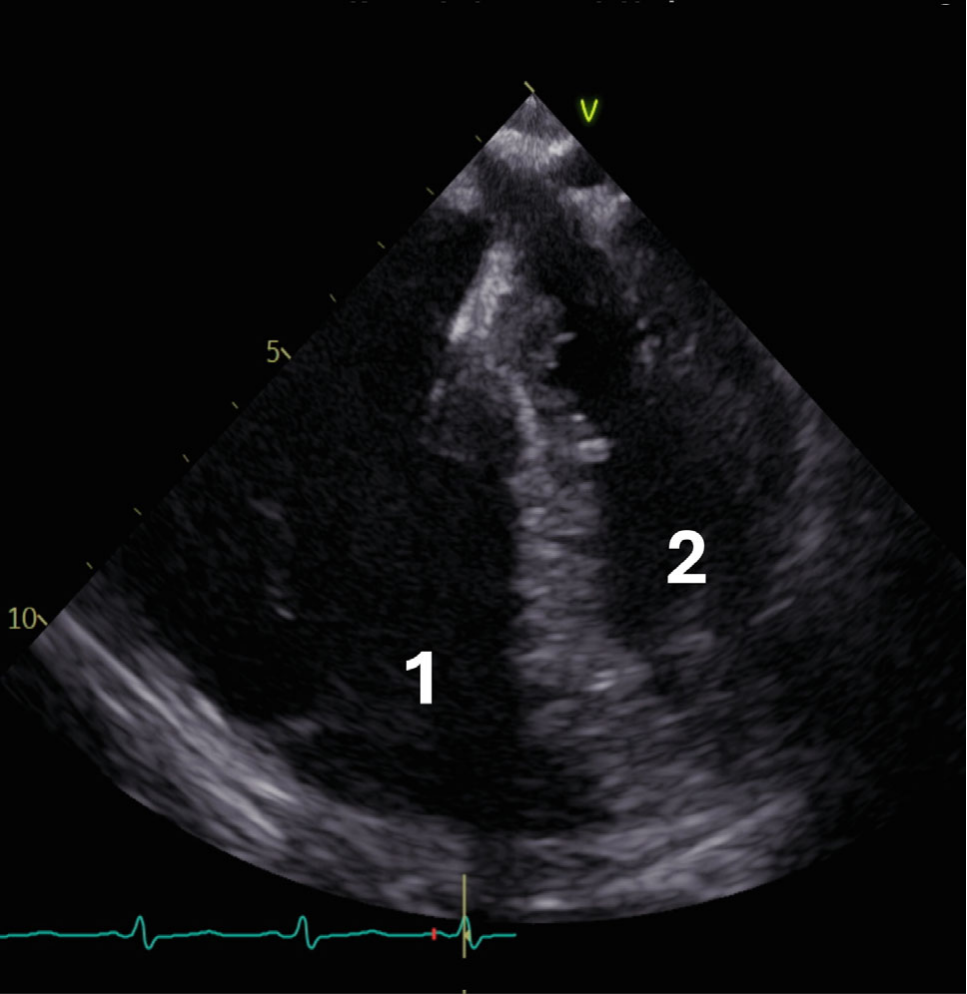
Transesophageal four‐chamber view with no sign of the right atrial or right ventricular (1) thrombus and the left ventricle (2).
